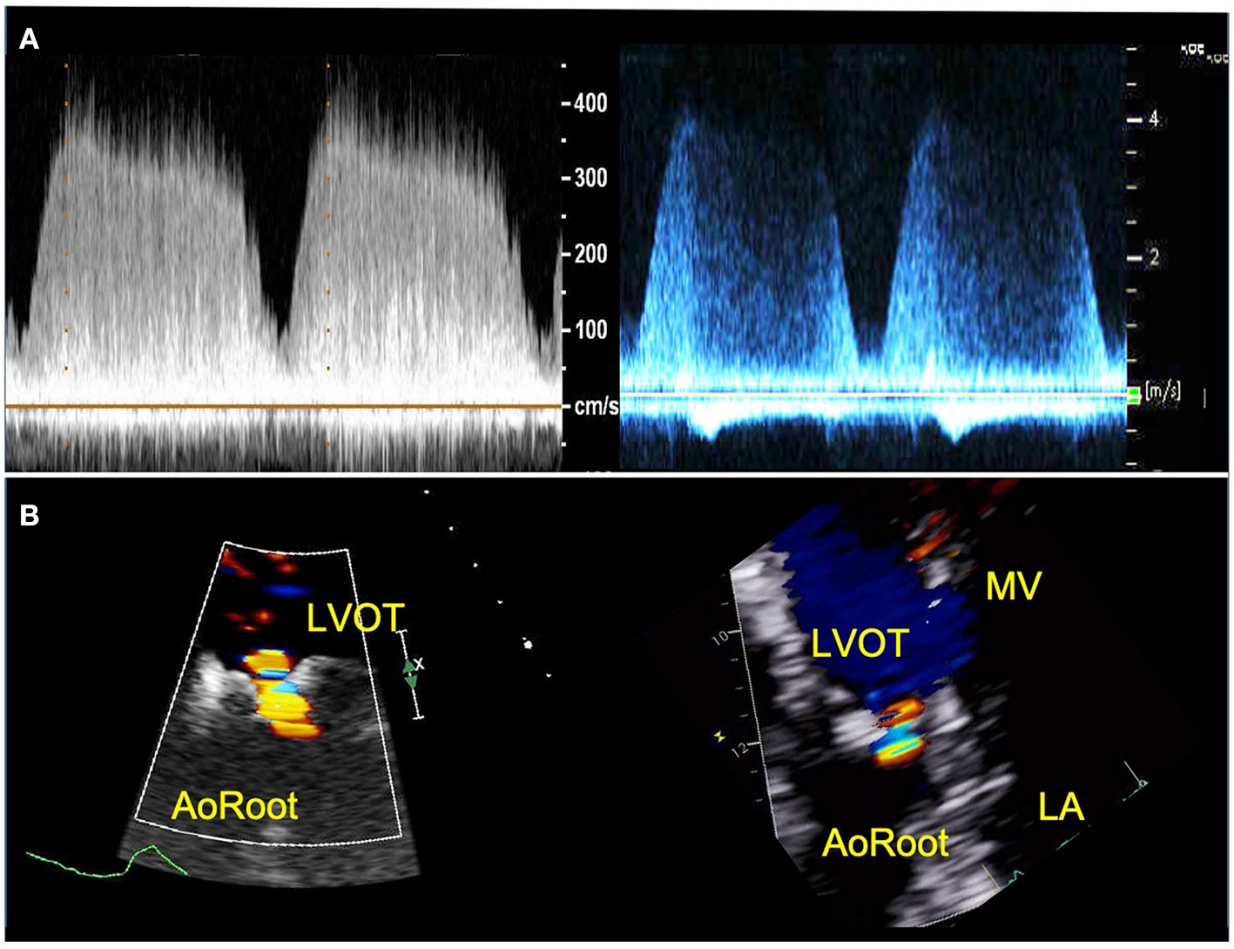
TTE images of AR from patient (right) and 3D printed model within the circulatory flow loop (left). (A) Continuous wave Doppler of AR flow demonstrating comparable profiles and peak velocities; (B) color Doppler images or the regurgitant AR flow, for vena contracta measurement. LVOT, Left ventricle outflow tract; LA, left atrium; MV, mitral valve.
